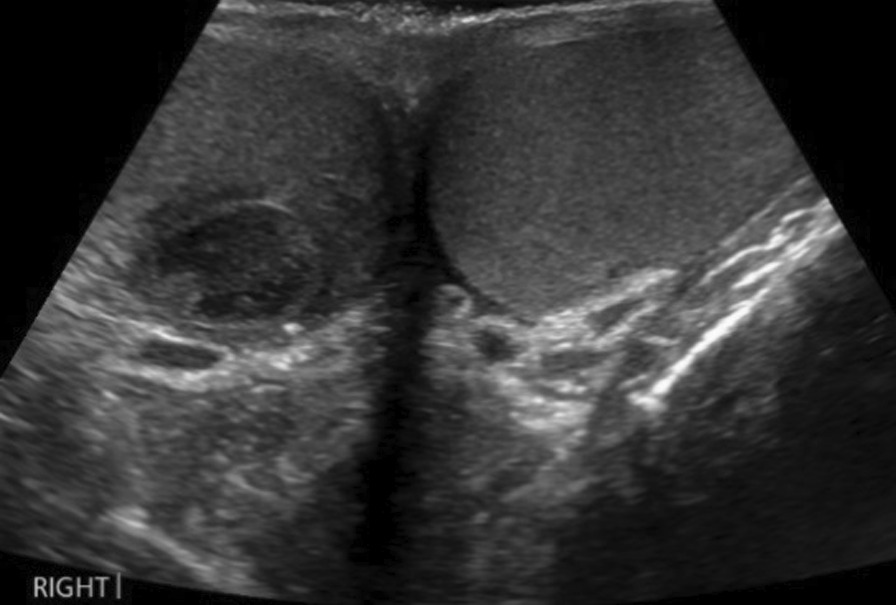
Testicular ultrasound showing hypoechoic lesion right testis and normal left testis
